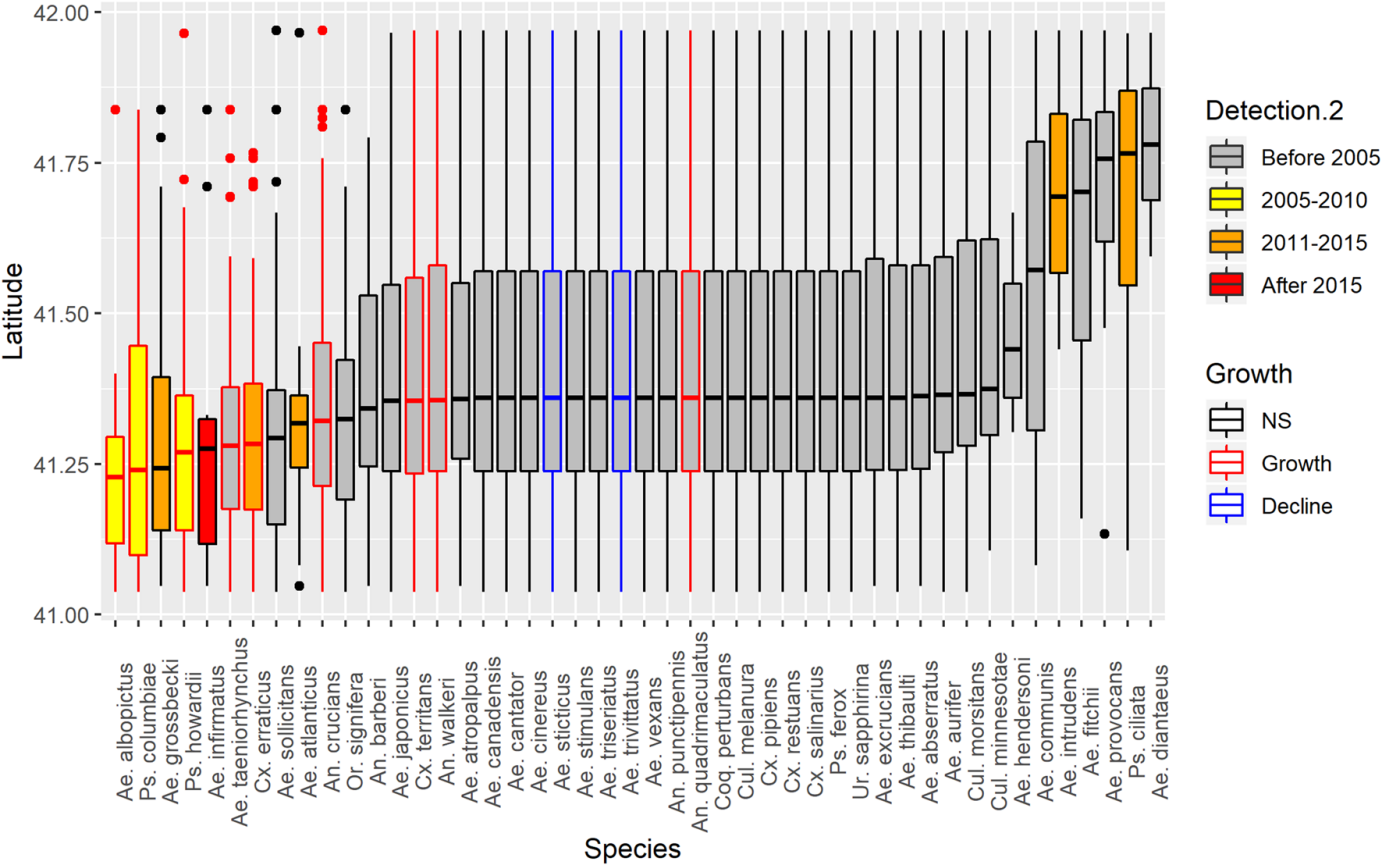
The latitudinal distribution of forty-six mosquito species collected in light traps across 87 surveillance locations in Connecticut, U.S. sampled with ground-level CO_2_-baited light traps from 2001 to 2019. Species are ordered by their average location of detection across all light trap collections in the CAES database. Fill colors represent the time period of first detection during standardized surveillance; border colors indicate statistical evidence of growth or decline in collections throughout CT; *NS* not significant. Species listed in Table 1 which are not listed here include *Aedes flavescens* and *Aedes spencerii* as there are only one collection of each species across all trapping effort. The figure was created in R V 3.6.3 using the *ggplot2* package.
